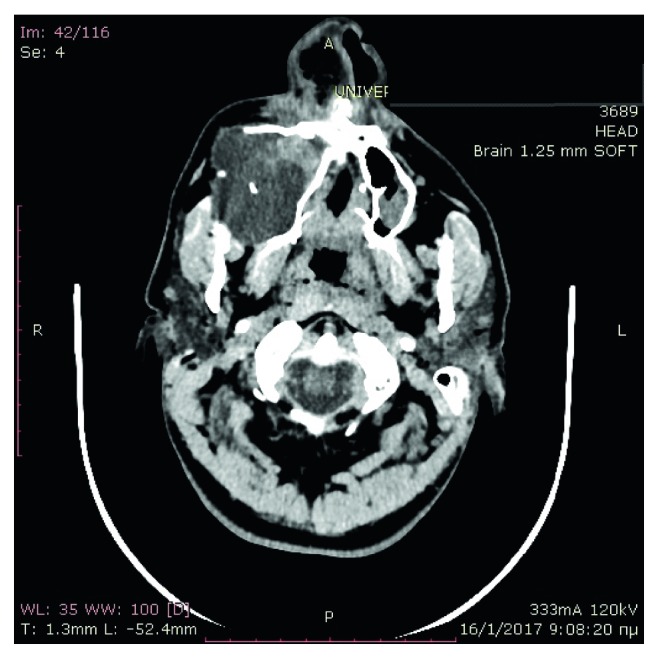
Preoperative CT, axial plane.
